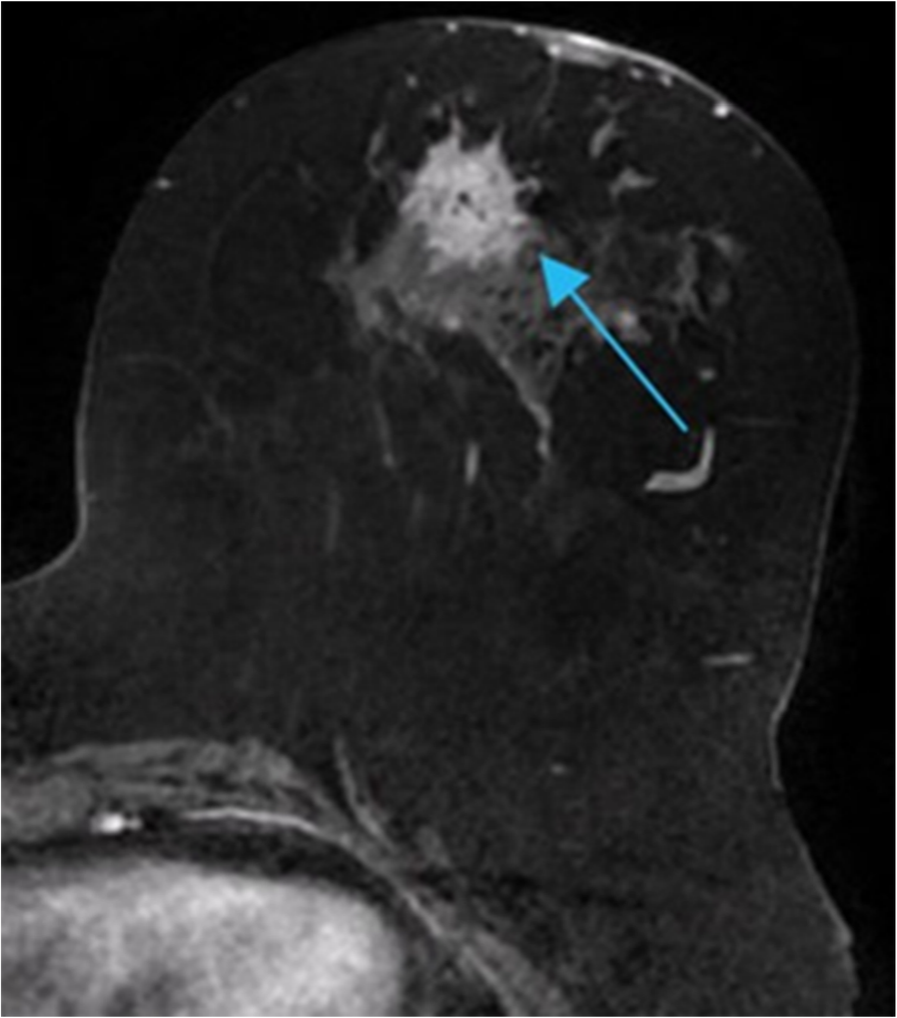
Axial fat-suppressed T1-weighted first post-contrast image of a breast cancer (arrow) where computer-extracted image phenotype (CEIP) sphericity is high and the radiologist assessed it as irregular for shape. CEIP and human-extracted image phenotype are discordant
